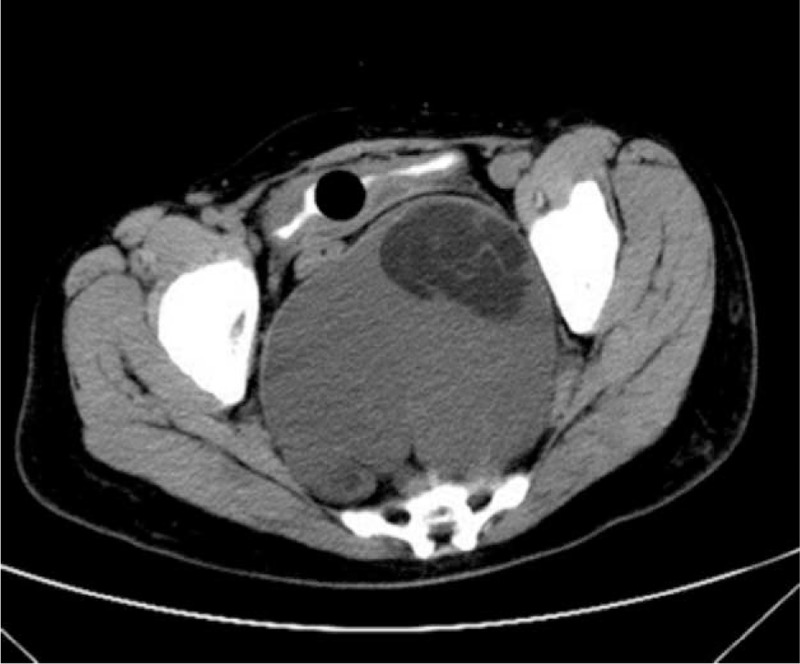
The CT image took in January 2015, 16 cm in size.
